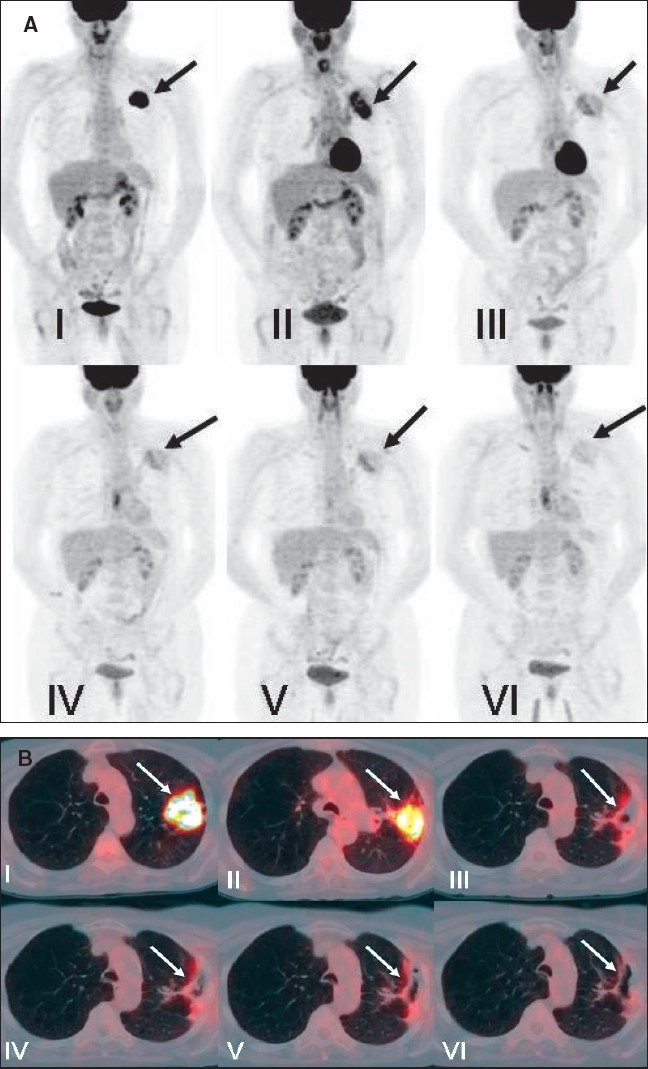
PET studies of a 58-year-old lady with left lung cancer. MIP images (A) and axial fused PET/CT images (B) of the left upper lobe mass of six consecutive PET scans are shown. The initial study (I) shows intense FDG uptake in the left upper lung neoplasm without loco-regional nodal or distant metastatic disease. A repeat study (II) 5 months after completing radiation therapy shows interval improvement with significant residual uptake. Subsequent studies (III-VI) 7 months, 11 months, 15 months and 22 months after completing therapy reveal gradual improvement in the intensity of uptake without evidence of residual/ recurrent disease
